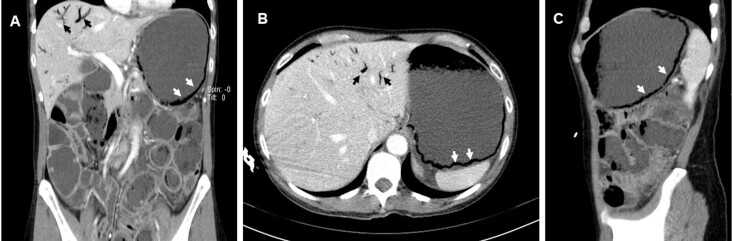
Abdominal-pelvic computed tomography (A - coronal; B- axial; C- sagittal) showing gastric pneumatosis (white arrow) and hepatic portal venous gas (black arrow).
